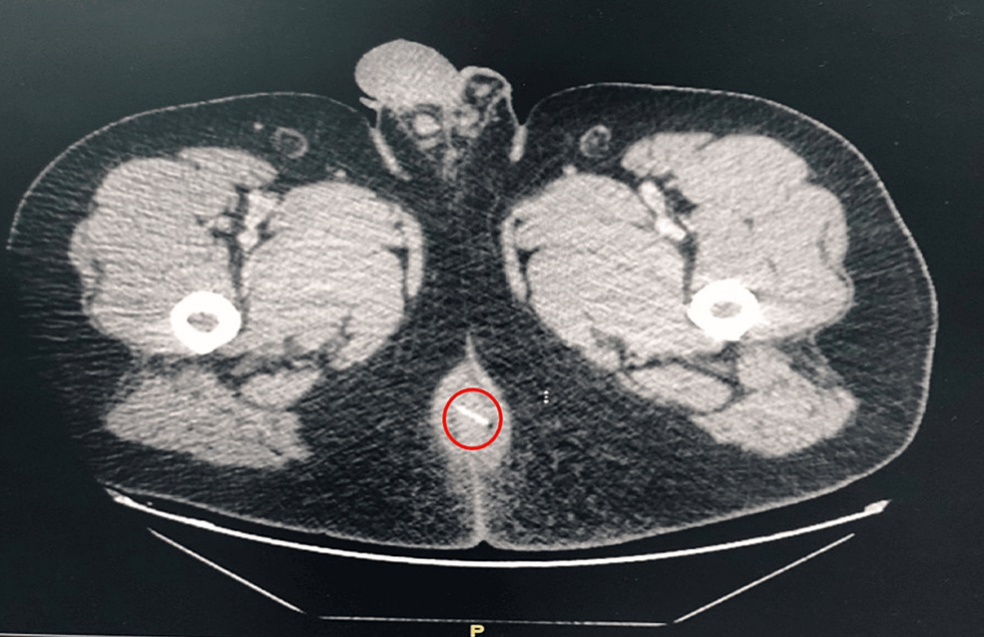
A CT scan of the pelvis (axial section) shows the linear foreign body (red cycle).
